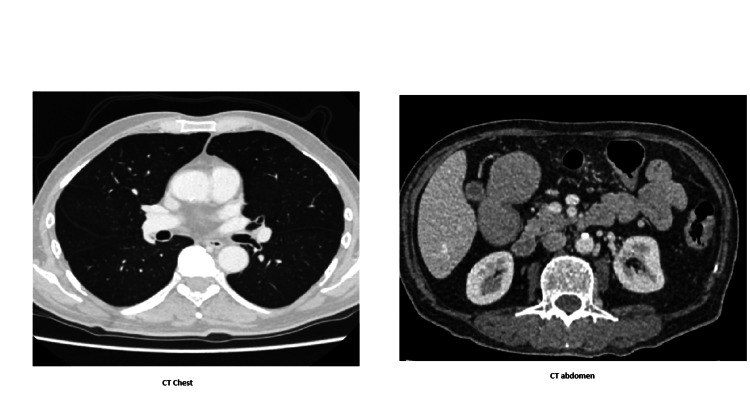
CT of the chest and abdomen showed normal findings.
